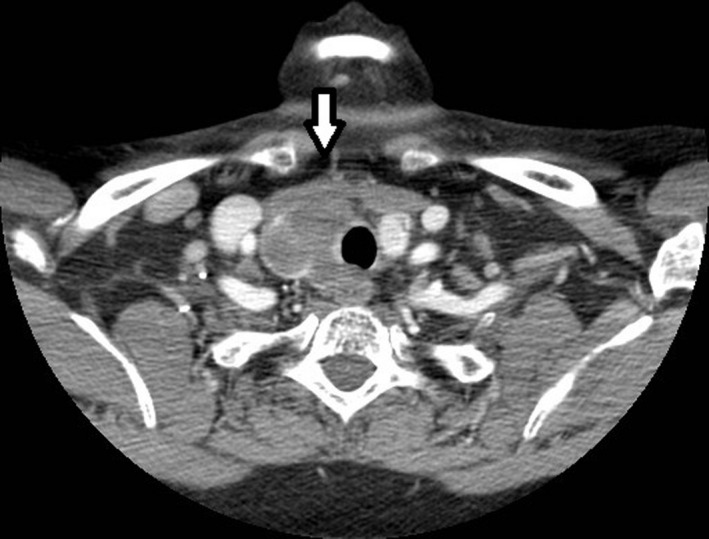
CT neck with solid‐cystic mass in the right thyroid lobe with variegated enhancement measuring 3.8 cm in largest diameter and no pathologic cervical lymph nodes
